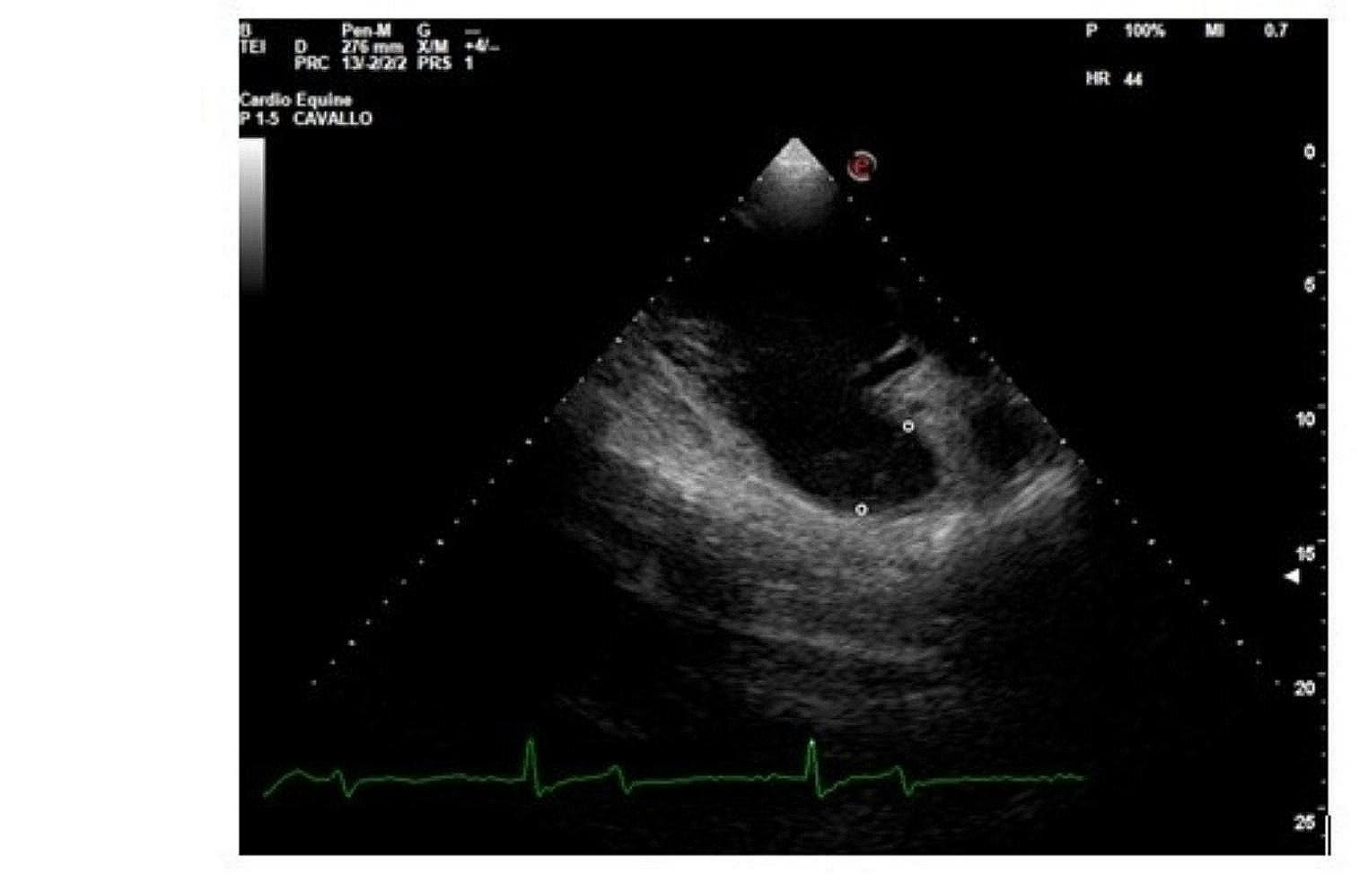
Right parasternal right ventricular inflow-outflow view showing the measurement of the pulmonary artery valve diameter - PAD (°) at end-diastole
